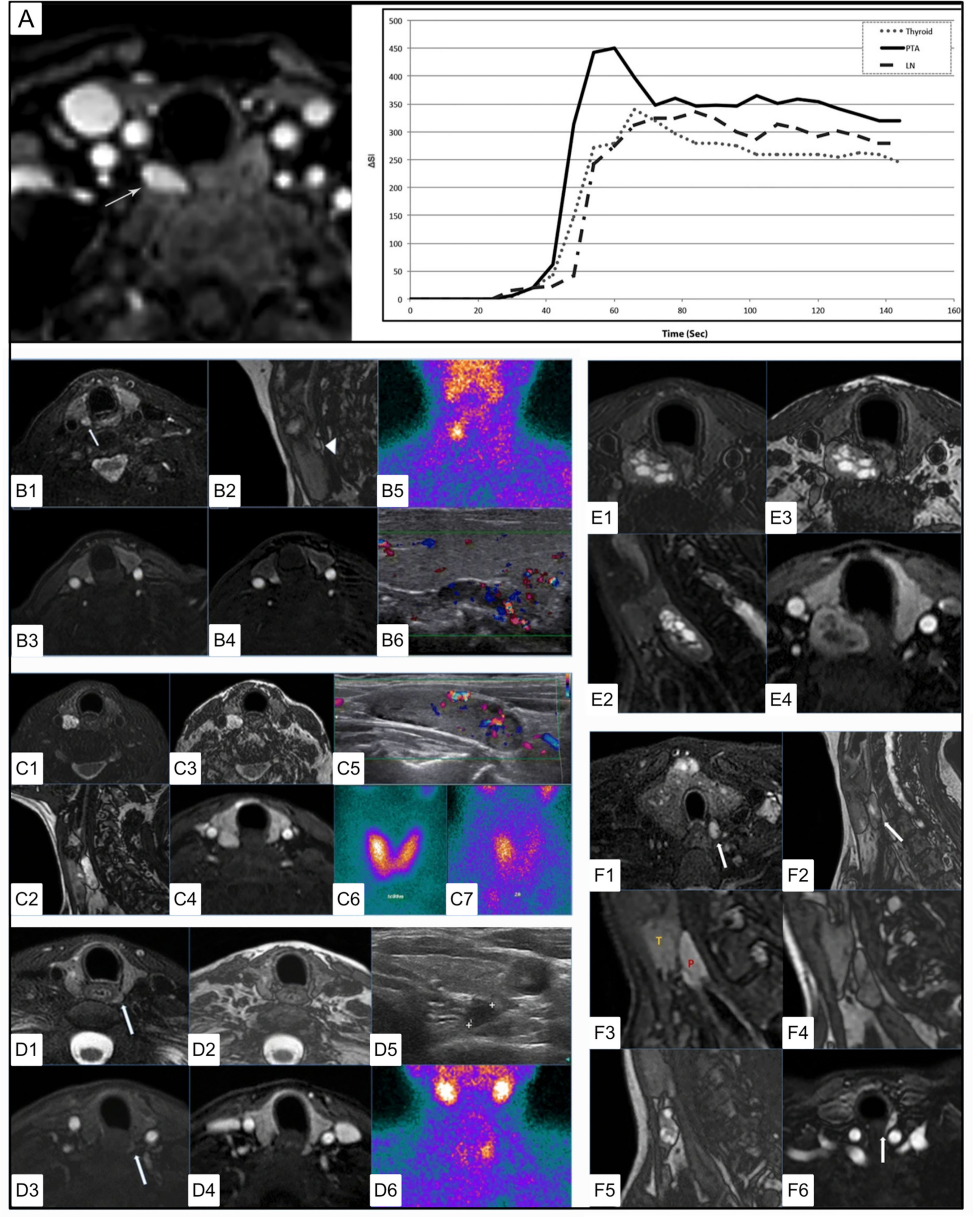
4DMRI imaging of parathyroid adenomas. (A) Axial arterial phase post contrast fat saturation T1 MRI of PA at the tracheoesophageal groove (white arrow) in a 47 yo F with primary hyperparathyroidism (PTH - 164 pg/mL, Ca2+ = 10.8). Graph depicts contrast-time curve analysis from ROI placed over the PA (solid), a lymph node (dashed), and the thyroid (dotted), showing relative faster time to peak (TTP), increased wash in and increased wash out values from the PA. (B) 4DMRI of PA posterior to the right thyroid mildly hyperintense relative to the thyroid on axial T2 fat saturation [(B1), white arrow] and separated by cleavage plane on sagittal out of phase imaging [(B2, white arrow] images with avid arterial enhancement greater than thyroid on T1 post contrast (B3) and T1 post contrast subtraction imaging (B4) and comparative coronal ^99m^Tc-sestamibi (B5) and ultrasound imaging (B6). (C) 4DMRI of PA posterior to the right thyroid gland with marked relative T2 fat saturation hyperintensity (C1), oblong appearance without reliable cleavage plane on out of phase imaging in sagittal (C2) or axial (C3) planes, and arterial enhancement similar to the thyroid gland (C4) and associated ultrasound (C5) and early (C6) and delayed (C7) phase ^99m^Tc-sestamibi. (D) Dynamic MRI of PA posterior to the left thyroid slightly hyperintense similar to thyroid on axial T2 (D1), with thin cleavage plane on axial out of phase (D2), with mild enhancement on early axial T1 post-contrast subtraction (D3) and similar to the thyroid gland on delayed axial T1 post-contrast subtraction (D4) and comparative ultrasound (D5) and coronal ^99m^Tc-sestamibi (D6). (E) 4DMRI of PA posterior to the right thyroid with mixed cystic and solid components causing mass effect on the esophagus displacing it to the left on axial (E1) and oblong appearance on sagittal (E2) T2 fat saturation, with cleavage plane on axial out of phase (E3), and partial enhancement similar to the thyroid gland on T1 post-contrast fat saturation (E4). (F) Summary of MRI features of PA including T2 fat saturation hyperintensity (F1), oblong appearance (F2), cleavage plane between the thyroid and PA (F3), which can be emphasized by india ink artifact on out of phase imaging (F4), ‘marbled’ appearance (F5), fast and strong enhancement on T1 post-contrast (F6). Reproduced with permission from: (A)
